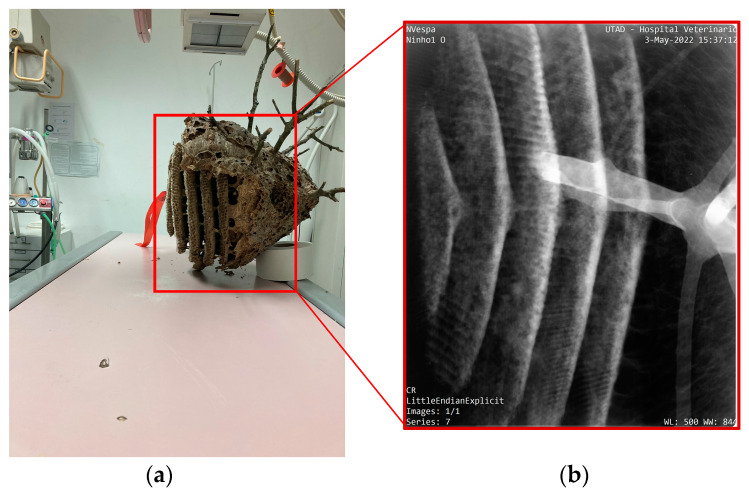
Radiographs of the AWN: (a) position of the nest during the X-ray scanning; (b) X-ray image of the comb layers.
